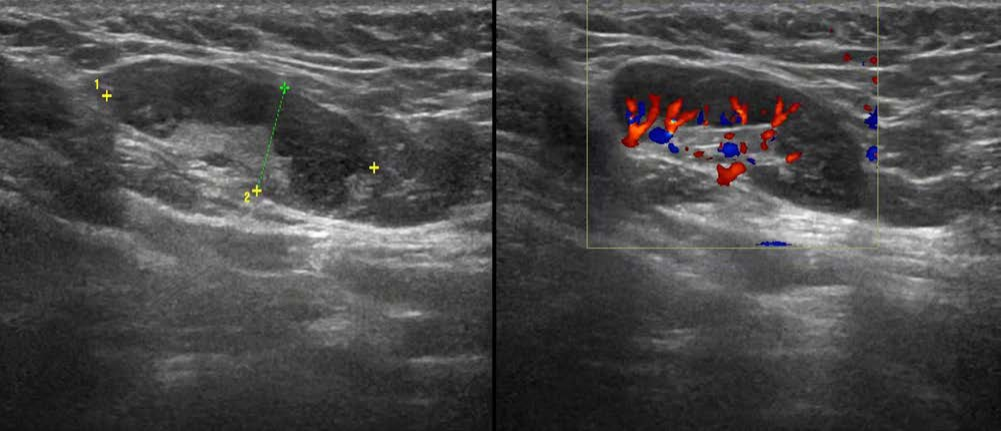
Transverse gray-scale ultrasonography (left), showing that the cortical echo of the right axillary lymph node was decreased and had uneven thickening. Color Doppler ultrasonography (right) showing hilar vascularity.
